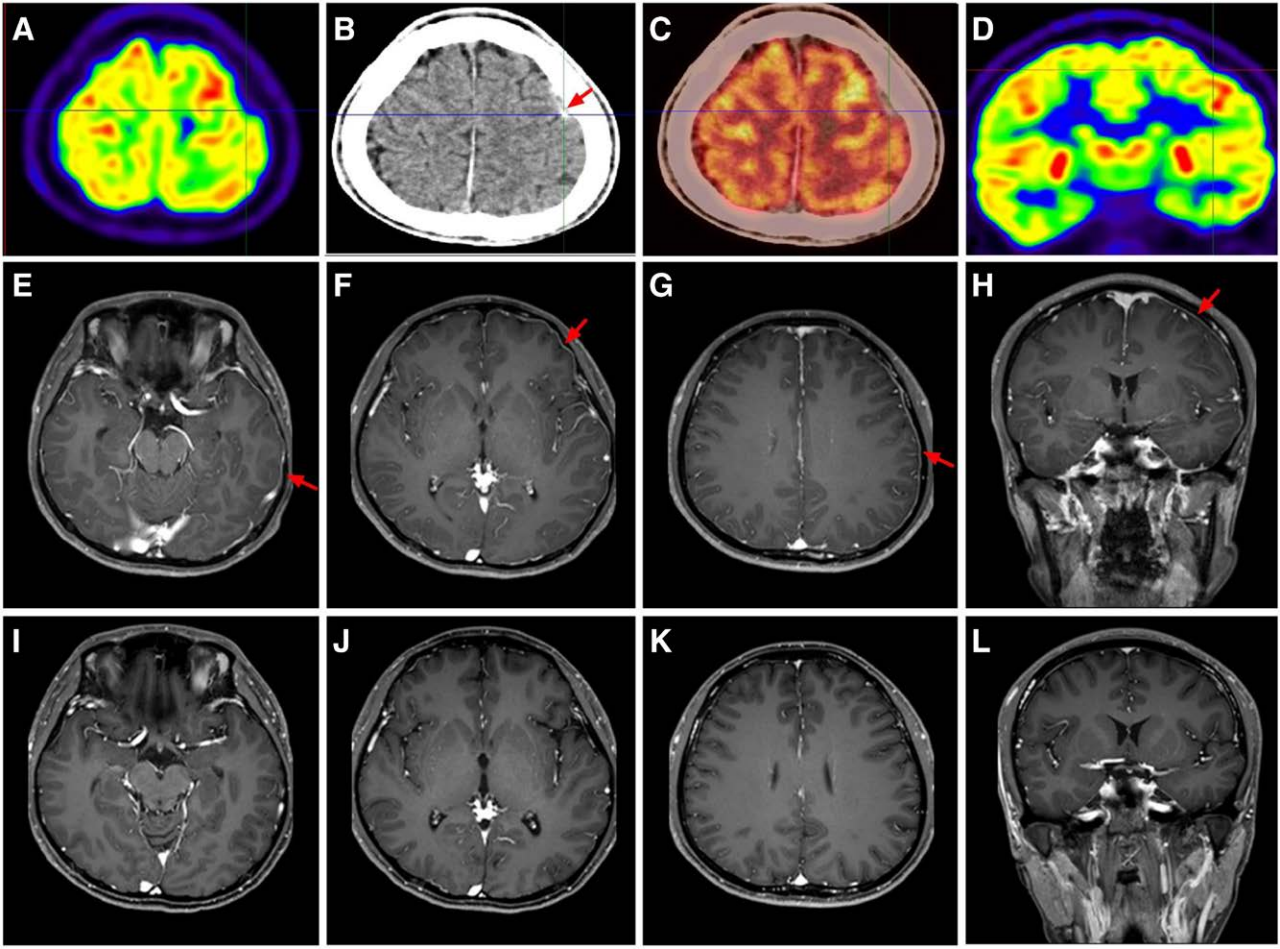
(A–D) In phase III, PET-CT of the brain revealed that the dura mater of the left frontal part was slightly thicker, metabolism was normal, and there were no obvious abnormalities in the brain parenchyma. (E–H) In phase III, the left dura mater was strengthened and thicker than the contralateral region in the enhanced brain MRI. (I–L) In phase III, the enhanced brain MRI showed that thickening of the dura mater was relieved after over 1 month of comprehensive treatment. PET-CT = positron emission tomography-computed tomography.
